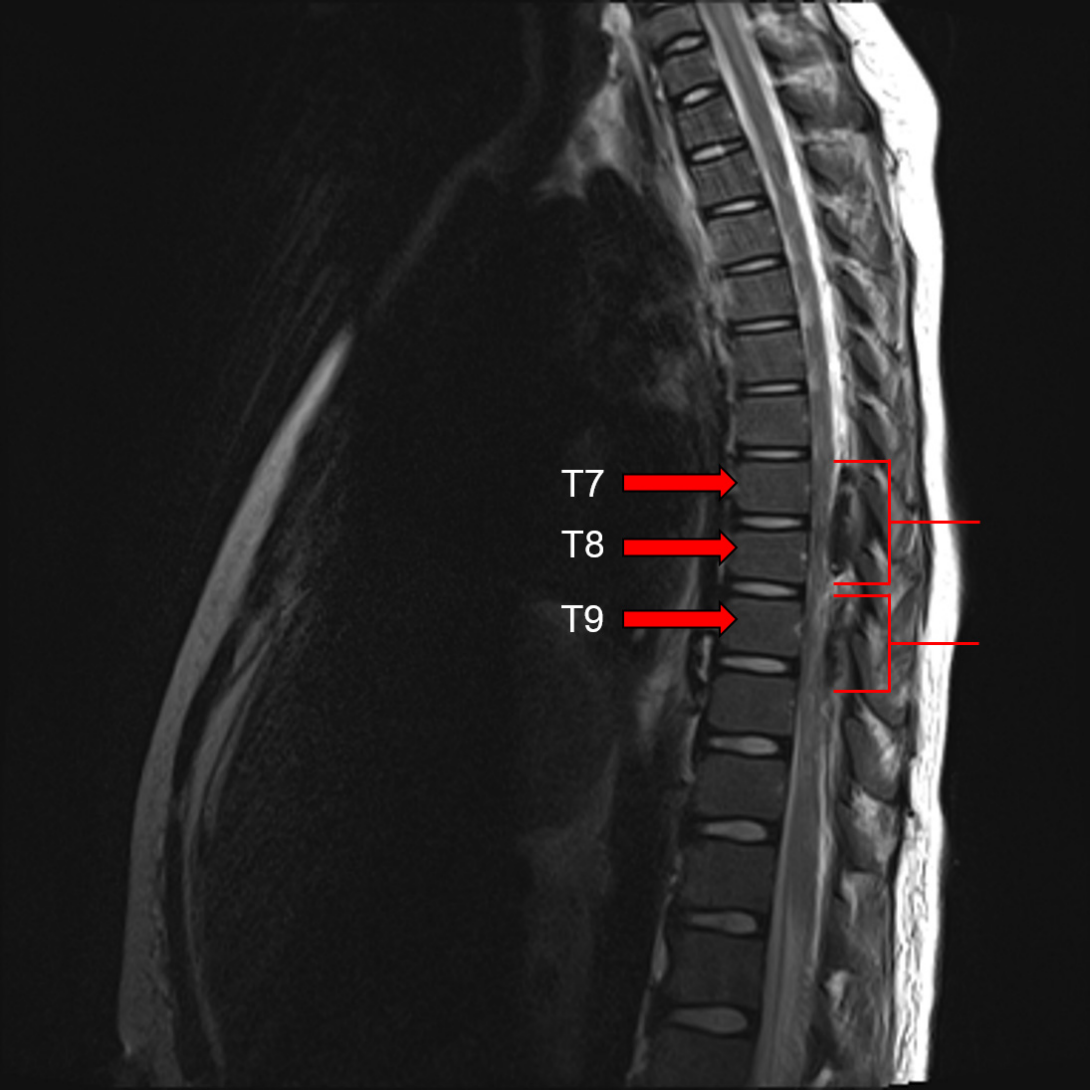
MRI T2 sagittal image is showing a mixed predominately hypo‐intense lesion within the spinal canal spanning T7‐T10.
